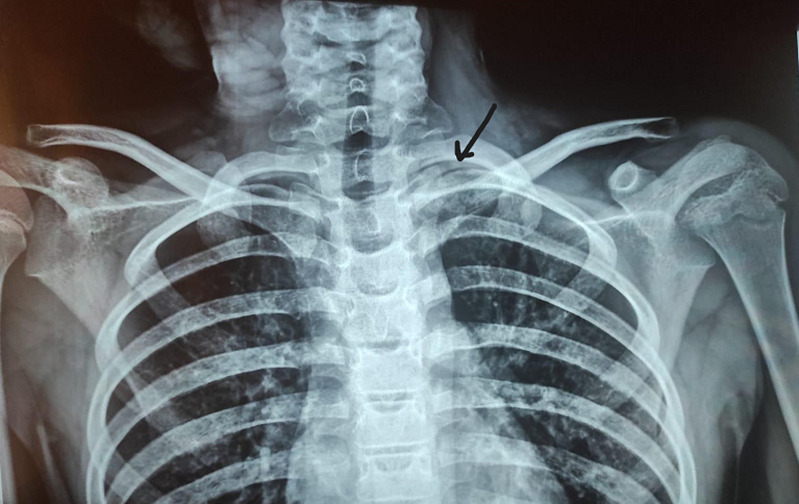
anteroposterior shoulders X-ray: osteolytic bone lesion with cortical disruption involving proximal part of left clavicle
